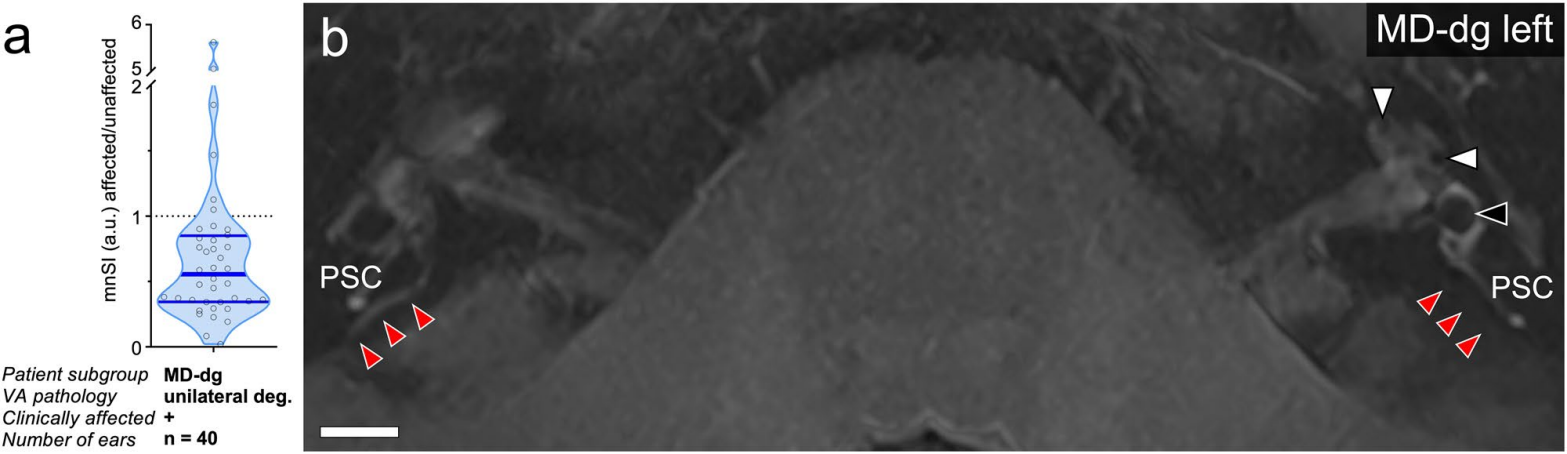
ES signal intensity in unilateral MD-dg cases. (a) The ratio of the normalized mean signal intensity (nmSI) between the affected and unaffected sides was determined after segmenting the ES signal (Fig. 1e) in axial MR images (3D IR sequence, maximal intensity projection). (b) Representative MD-dg patient with a clinical diagnosis of left MD and endolymphatic hydrops in the left cochlea (white arrowheads) and saccule (black arrowhead). Red arrowheads indicate the ES signal with its most distal (posterior) extension. *PSC* posterior semicircular canal. Scale bar 10 mm.
